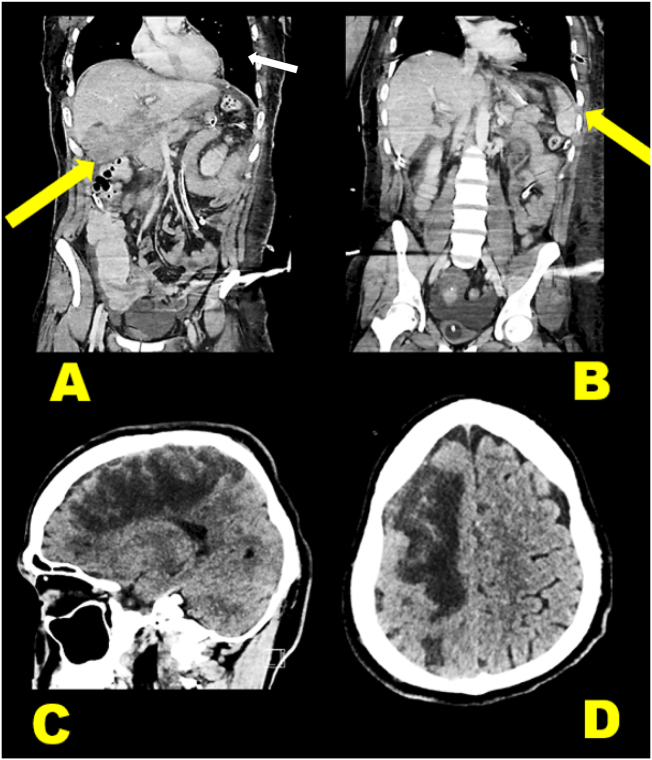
Major CT findings, A; Grade-II splenic injury (arrow), B; Grade-IV Liver injury (arrow), C & D; Rt. Fronto-parietal cerebral haematoma.
